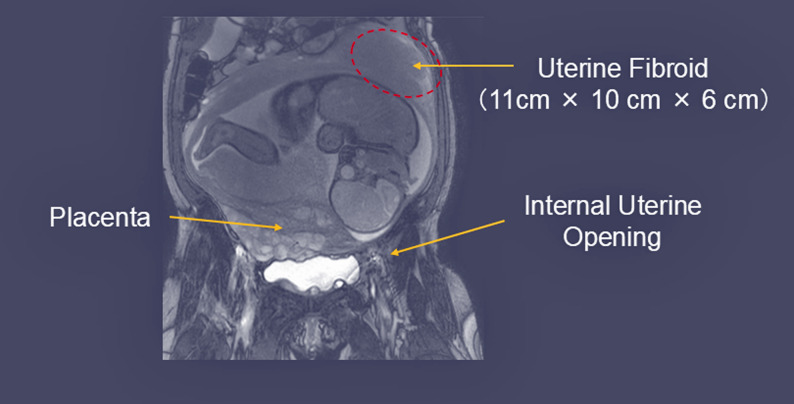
MRI T2-weighted image of the uterus at 34 weeks and 3 days of gestation. MRI, magnetic resonance imaging
